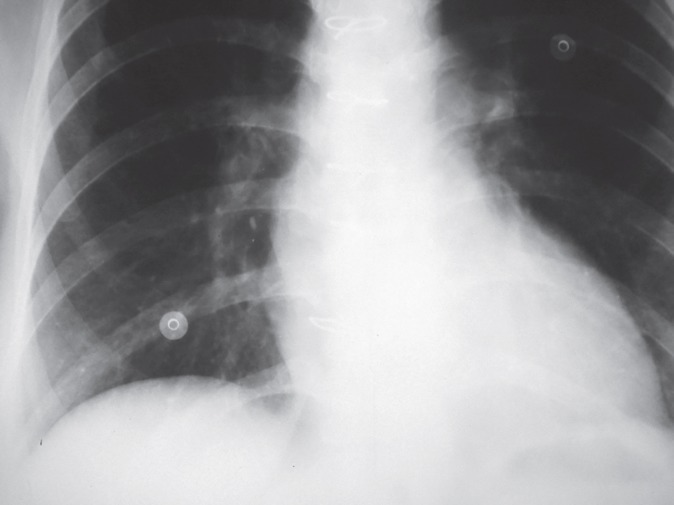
Chest x-ray showing infiltration in the right-lower and middle lobes
